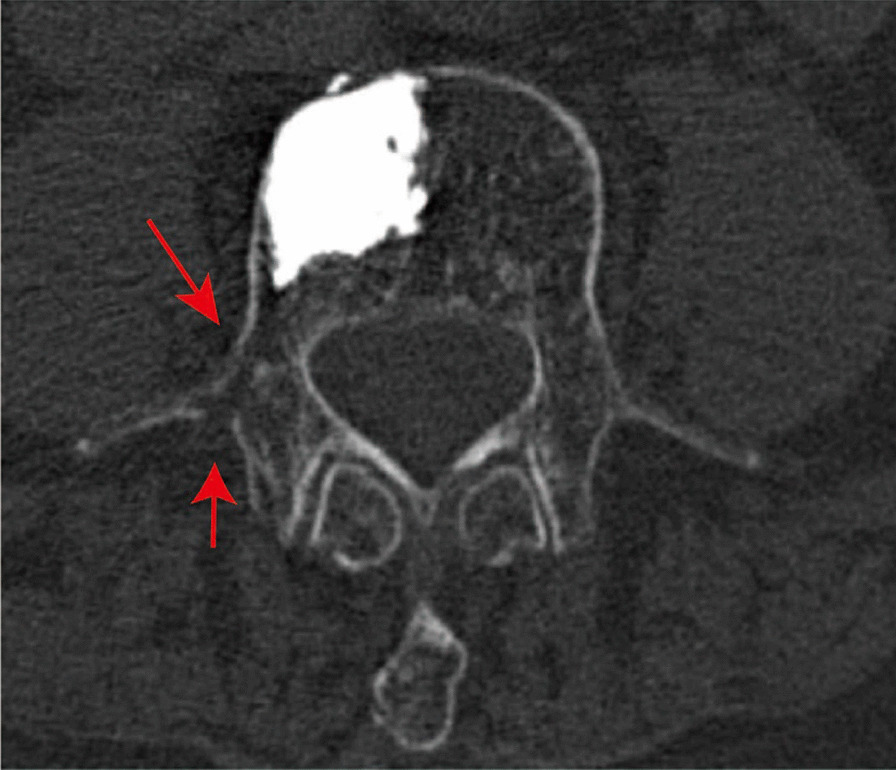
Extra-facet puncture PVP surgery with transverse process fracture. Female, 64 years old, who suffered L4 vertebral compression fracture and accepted extra-facet puncture PVP surgery. The postoperative CT transversal image showed L4 transverse process fracture
